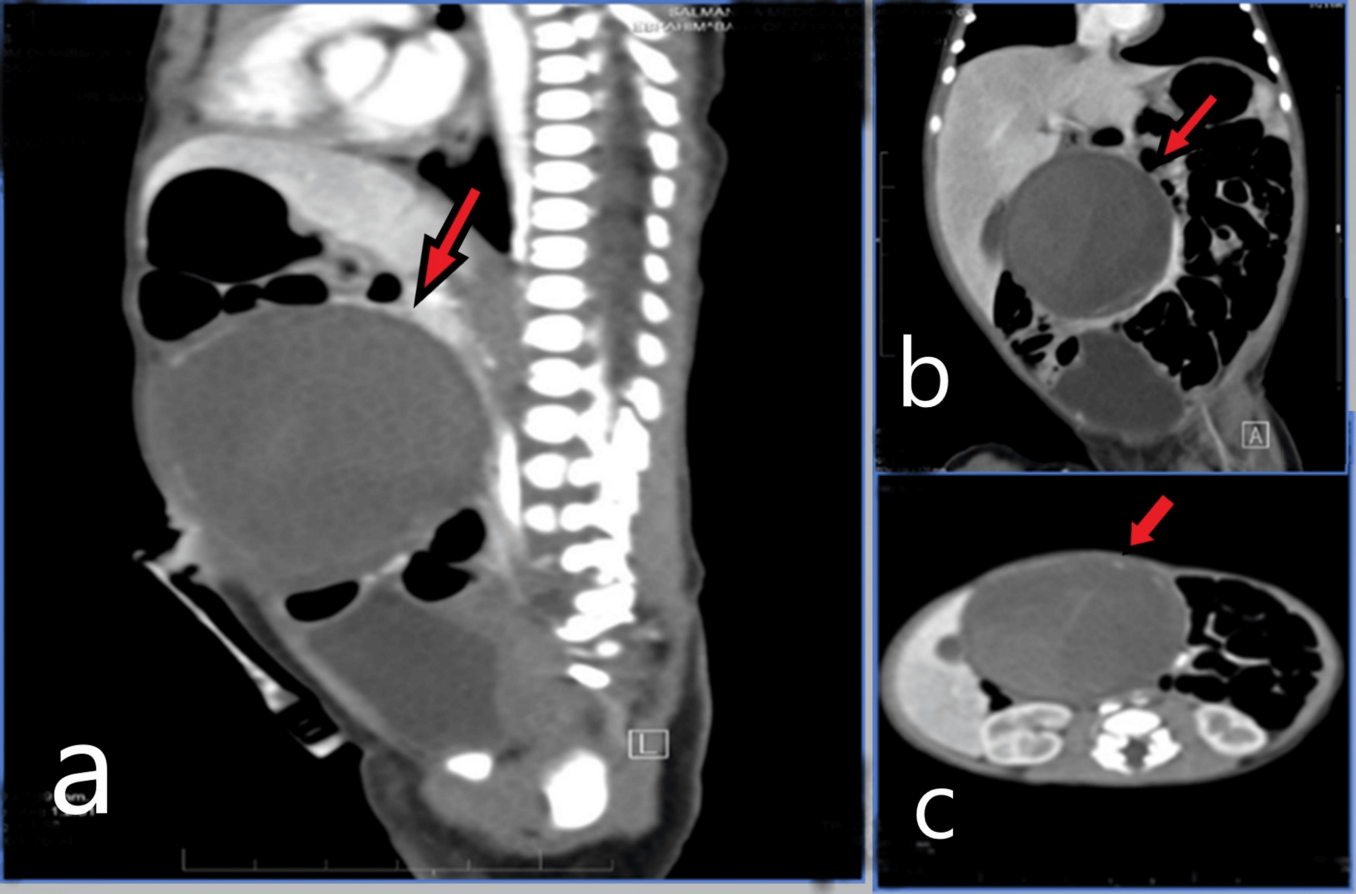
Cross-sectional CT images a. Sagittal image; b. Coronal image; c. Axial image The cuts demonstrated the complex cystic lesion, which was extending from the porta hepatis up the umbilicus anteriorly, which was exerting a mass effect on the adjacent structures. There was no invasion or encasement to the adjacent structures and the major abdomen organs were grossly normal.
